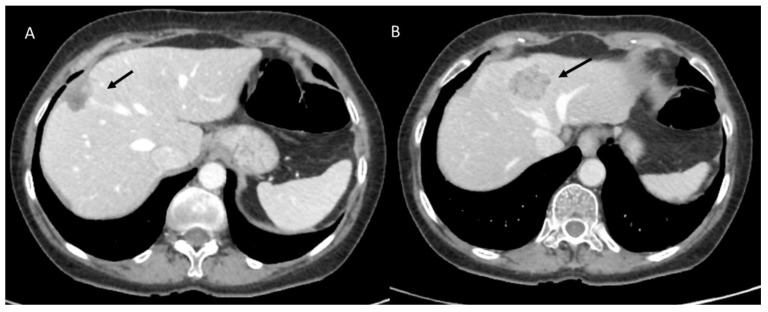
CT assessment in colorectal metastasis-resected patient (A). In (B), arrow shows a new lesion.
